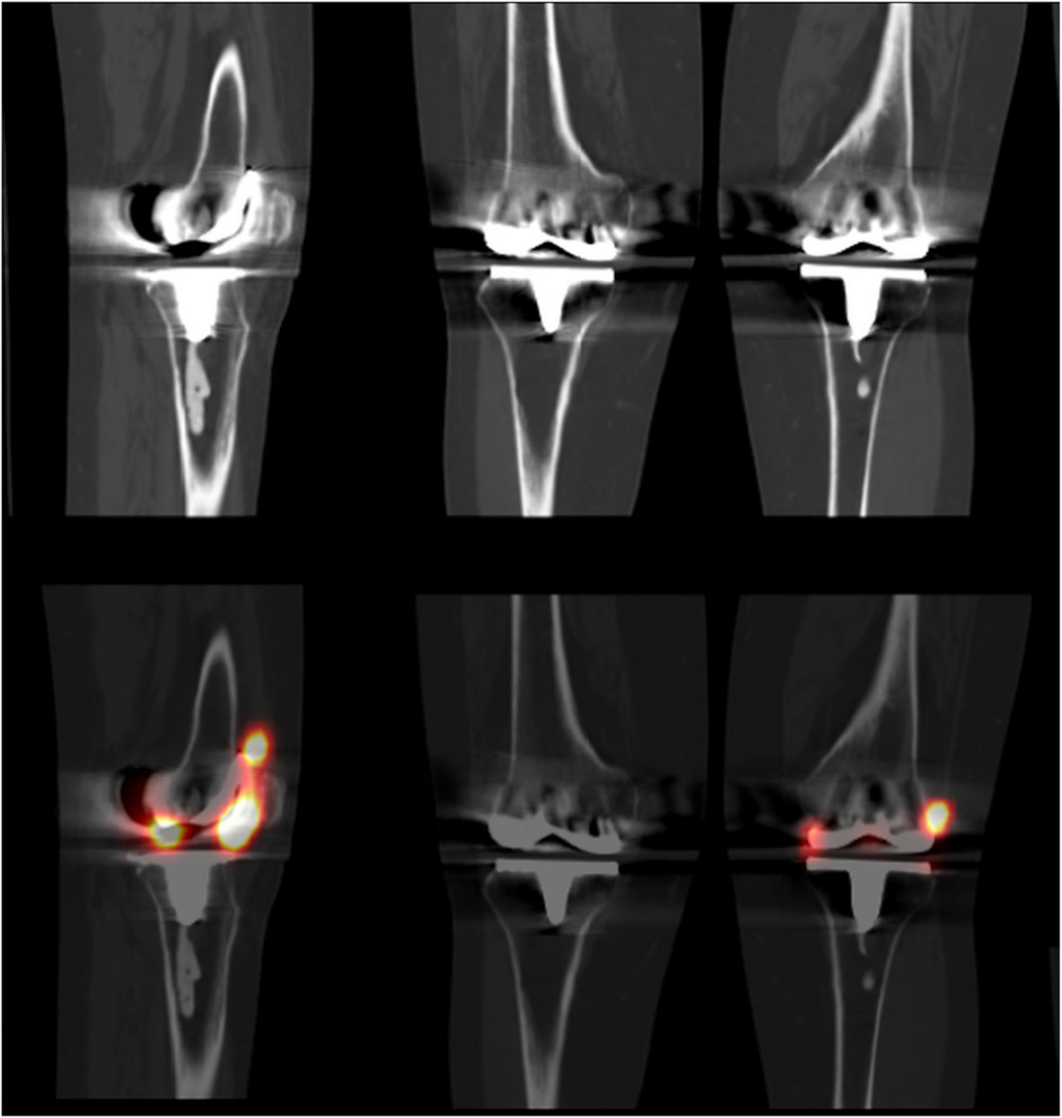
Normal left knee total arthroplasty. Sagittal (left) and coronal (right) imaging with CT (top row) and fused SPECT/CT (bottom row) images showing normal tracer activity within the joint space
